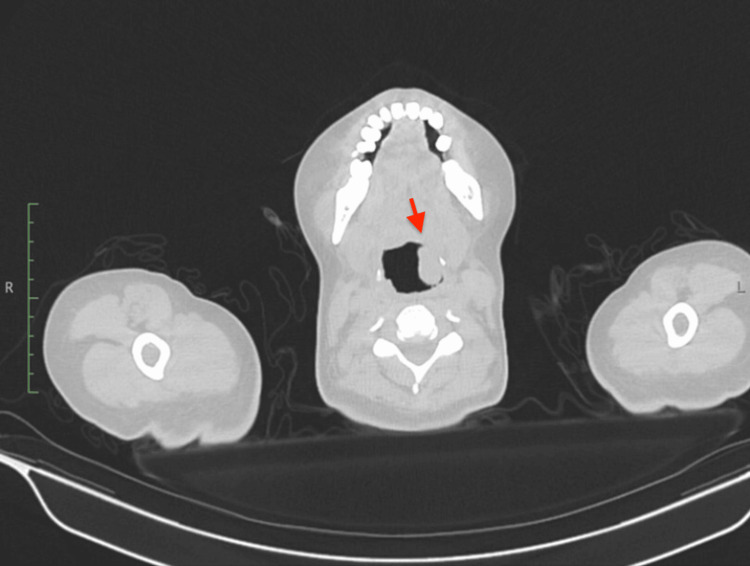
Contrast-enhanced CT of the head and neck, axial plane A concentric, asymmetric 18 x 49 mm mass arising from the left oropharyngeal mucosal wall resulted in 60% airway stenosis (red arrow).
